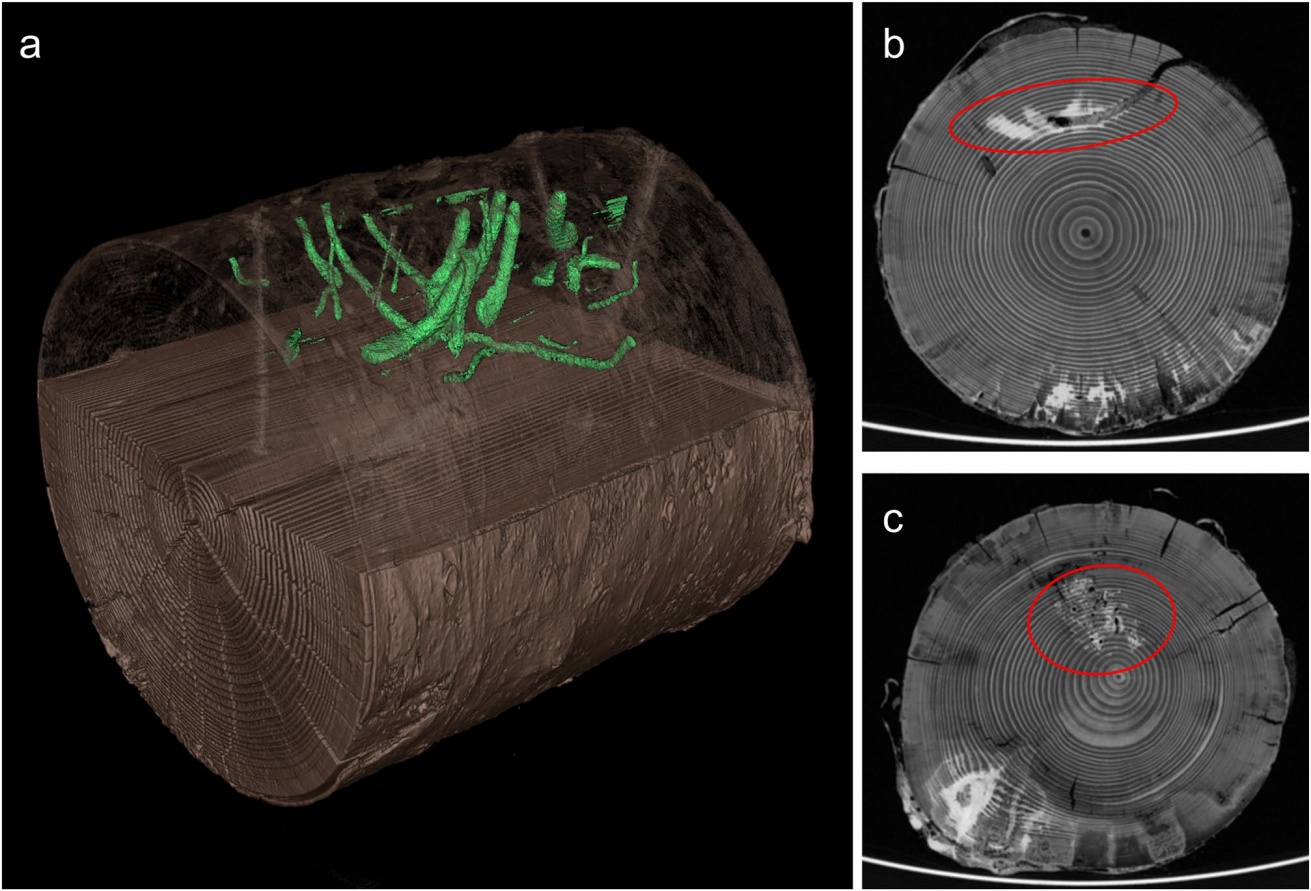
Computed tomography allows spatial analyses of beetle and fungal activity patterns and species interactions. (a) Shows the 3-D structure of beetle tunnels in a deadwood log reconstructed from automatically detected tunnel structures (generated with X-AID 3D volume viewer). In (b) and (c), areas of fungal decay (white) are located close to beetle tunnels indicating that both taxa are closely interacting.
